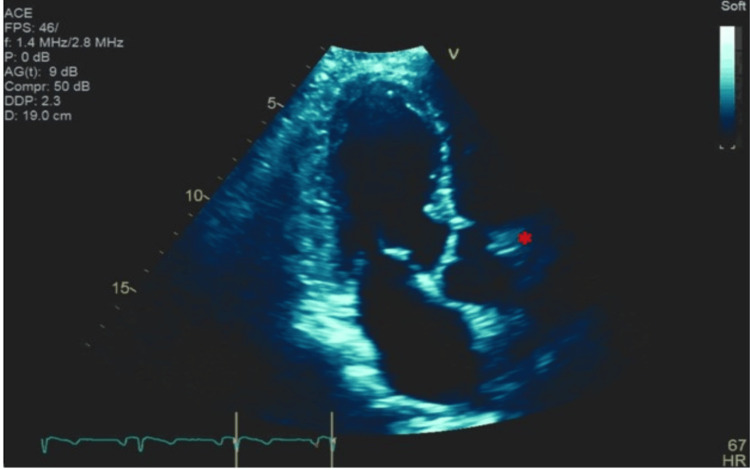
Transthoracic echocardiogram in a three-chamber view Transthoracic echocardiogram (TTE) in three-chamber view. At the level of the right coronary sinus, a tubular, echodense structure can be seen arising from the right coronary sinus, which protrudes into the aortic lumen at approximately the 1 o’clock position (marked with red asterisk).
